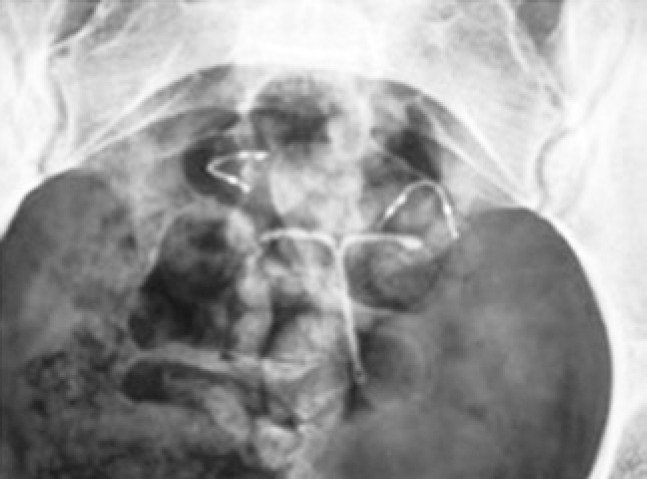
Pelvic x-ray showing intrauterine system and microdevices in tubes
